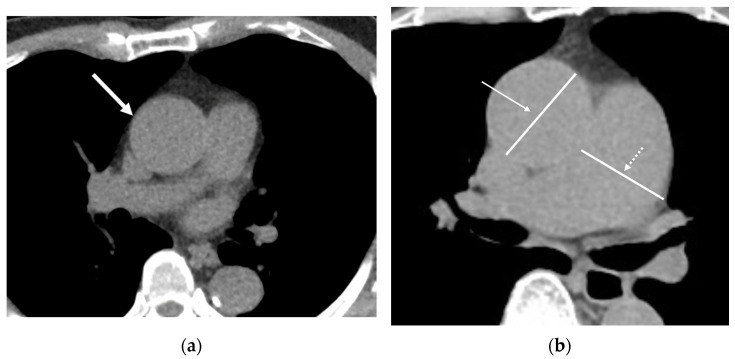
The incidental findings of abnormalities in the cardiovascular system on low-dose computed tomograph (LDCT). (a) An axial LDCT of a 64-year-old male with hypertension demonstrates a dilated ascending aorta (AA, arrow), which measures 48 mm in diameter. The size of the AA, though not exceeding the aneurysm threshold of 50 mm, warrants further assessment and a potential referral to a cardiologist due to its size exceeding 42 mm. (b) An axial LDCT of a 71-year-old female demonstrates an ascending aortic dilatation (arrow) measuring 45 mm and a dilated main pulmonary artery (MPA, dashed arrow) measuring 37 mm. MPA dilation, exceeding 31 mm, is potentially associated with pulmonary arterial hypertension and warrants further evaluation by a cardiologist or pulmonologist.
